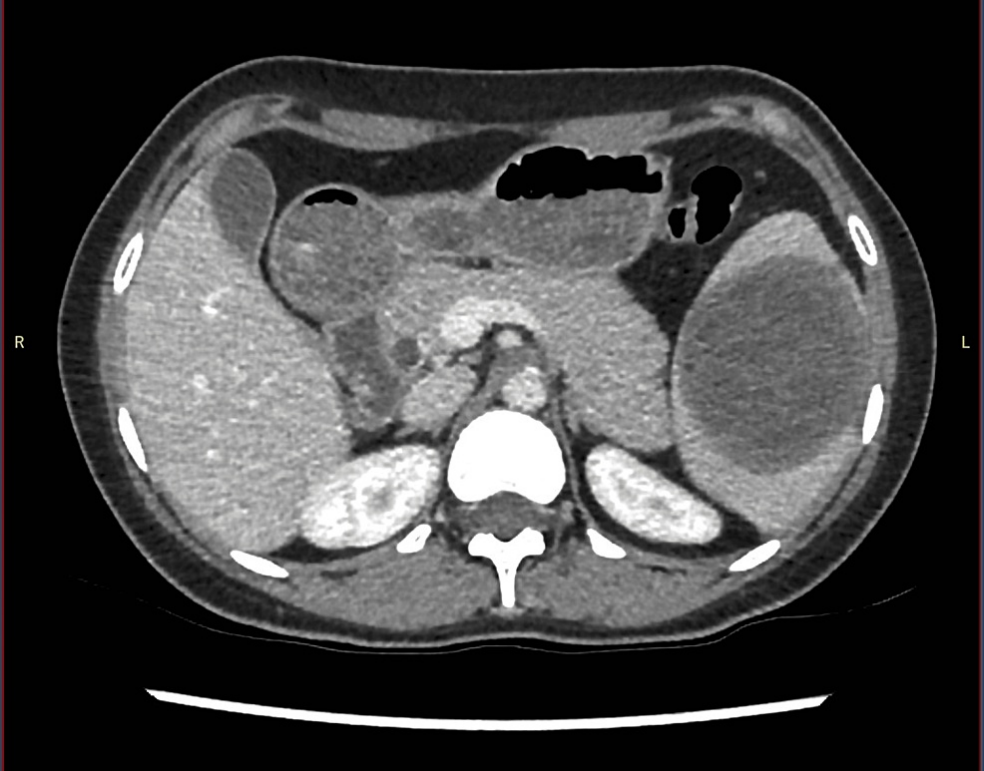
Serial CT imaging showing an interval decrease in the size of the cyst due to pharmacological interventions
